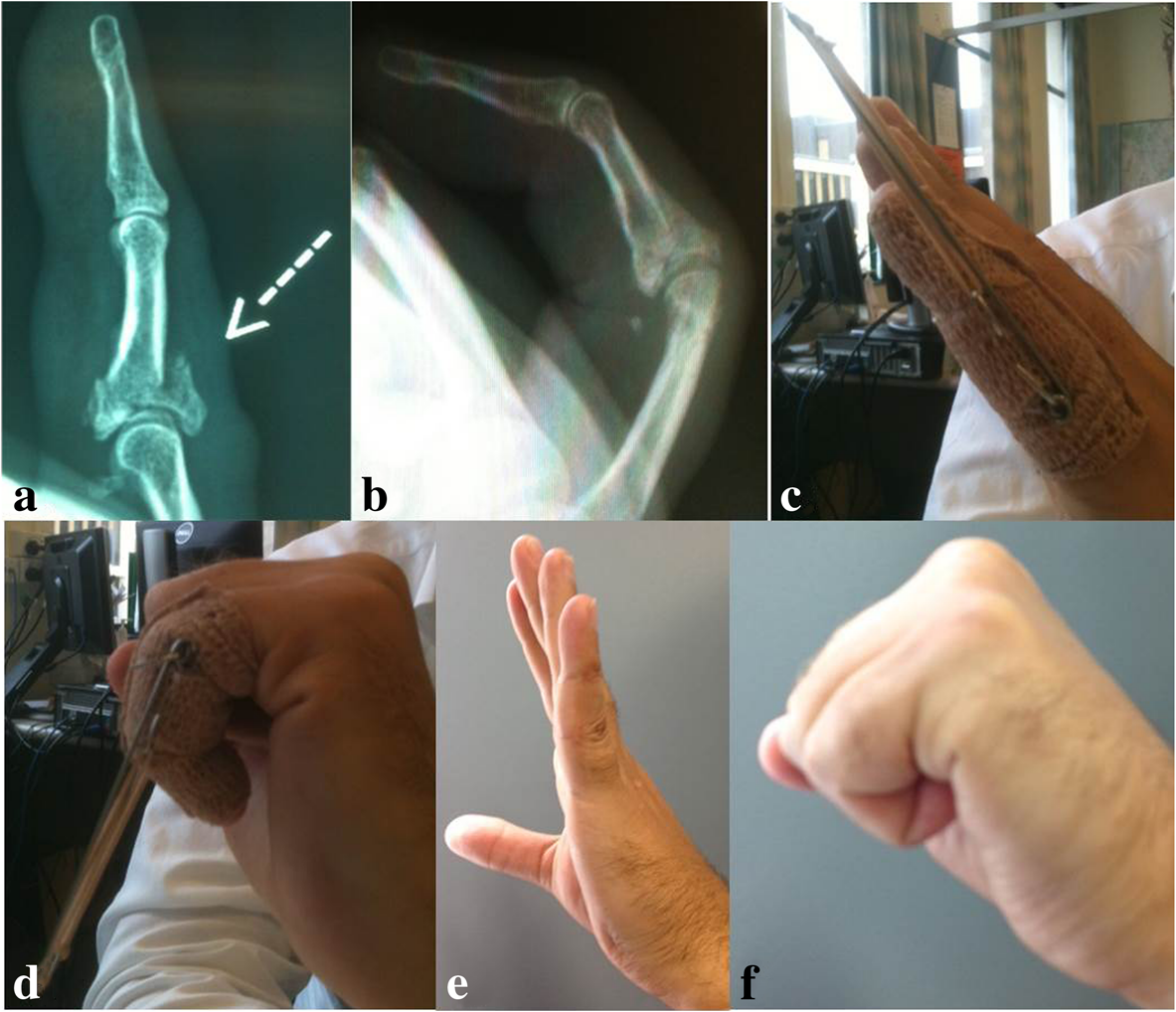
A patient with left little finger pilon type proximal interphalangeal joint (PIPJ) fracture. a Pre-operative X-ray. b X-ray post removal of distraction frame demonstrating satisfactory fracture reduction and healing. c, d Early active motion with distraction frame. e, f Excellent range of motion (ROM) achieved
